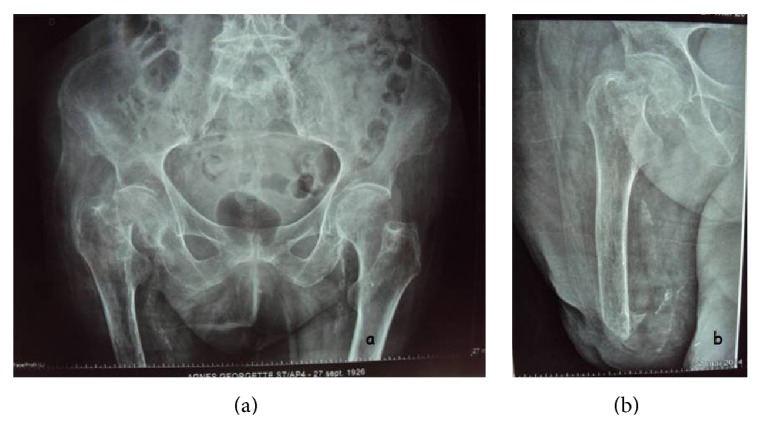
The X-ray of the pelvis and the hip (an anteroposterior X-ray) showing a fracture of the femoral neck.
